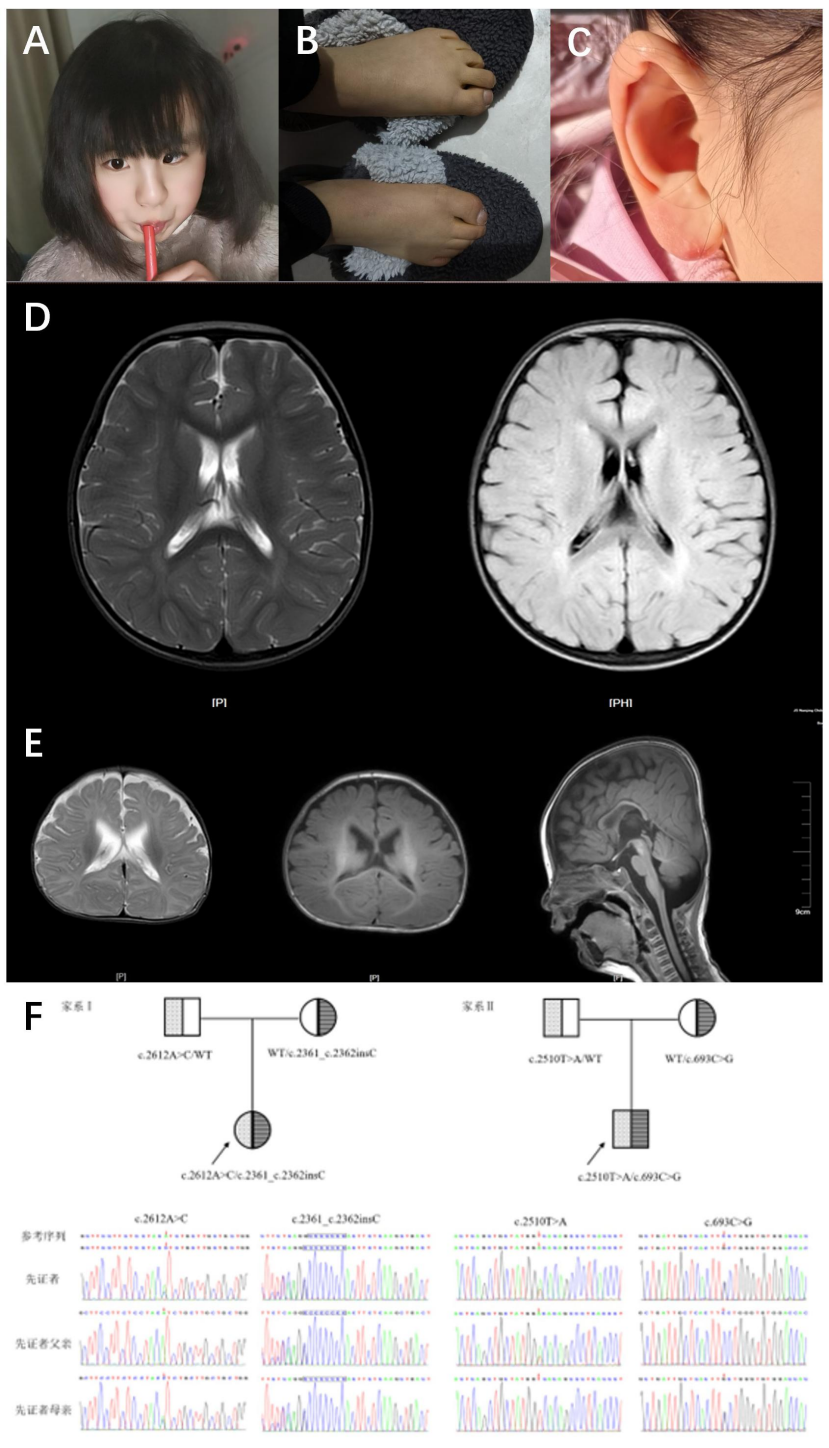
Typical features and brain MRI in 2 cases with HPIDS2. (A) Patient 1 (9 years 3 months) facial photo, showing left eye esotropia. (B) Patient 1 (9 years 3 months) foot and toe photo, showing thickened toes, yellow skin, and excessive skin keratinization. (C) Patient 1 (9 years 3 months) ear photo, showing ear malformation. (D) Patient 1's brain MRI shows the following findings: widened extra-axial spaces. (E) Patient 2's brain MRI shows the following findings: 1. Bilateral enlargement of the lateral ventricles, with widened extra-axial spaces in the temporal and frontal regions on both sides. 2. Slight delay in myelination. (F) Sanger sequencing chromatograms of PIGO gene mutations in two HPIDS2 patients from the family. The mutated bases are indicated by red arrows and blue boxes.
